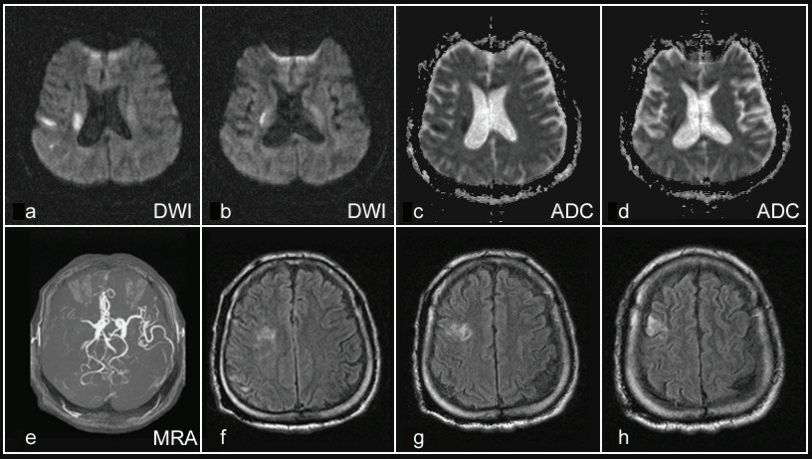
Imaging findings of the patient. Axial diffusion-weighted imaging (DWI) of brain MRI reveals several small acute infarcts involving the frontal and parietal lobes, right corona radiata and dorsal putamen shown with bright signal in the distribution of the right MCA territory and in the territories of the posterior watershed area compatible with the area of the apparent diffusion coefficient (ADC) reduction (a–d). The 3D time-offlight (TOF) MR angiography demonstrates abrupt disruption in mid-segment of M1 of right MCA (e). Long TR sequences demonstrate high signal abnormality centered in the right temporal, occipital, and middle frontal lobes suggesting old ischemia or infarct on the right cerebral hemisphere (f–h).
